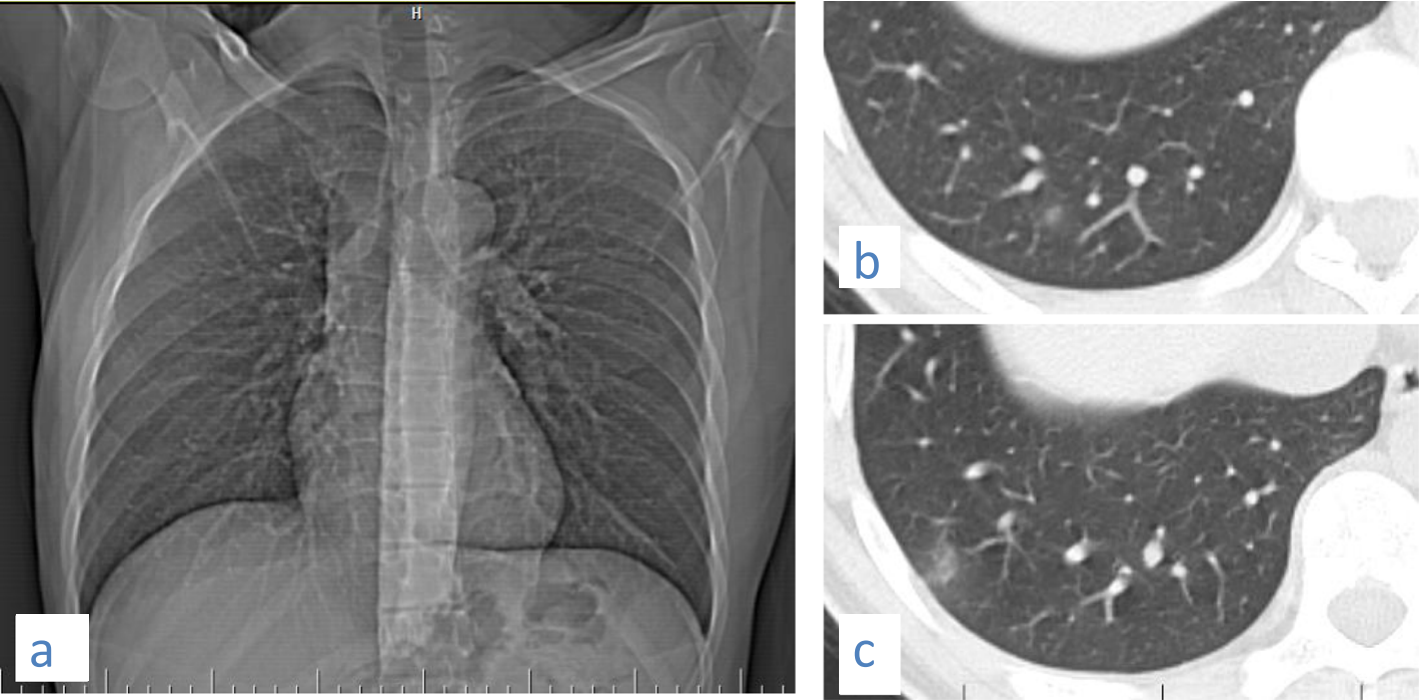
Male patient 28 years old with COVID-19. CT scout image (a) shows no abnormality while magnified axial CT image (b) shows right lower lobe peripheral rounded ground glass opacity, and (c) shows a subpleural nodule
